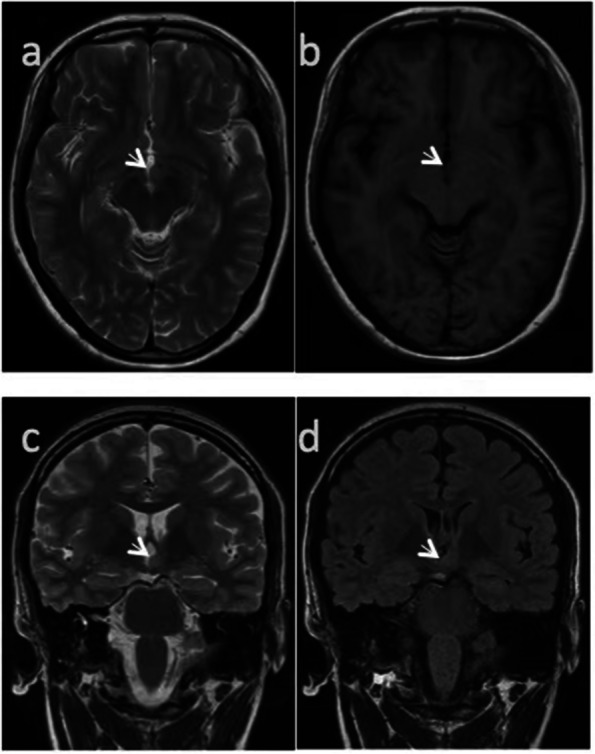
Cranial magnetic resonance imaging (MRI). Axial T2WI (a), T1WI (b), coronal T2WI (c), and T2 FLAIR (d) all showed presence of HH in the left side, with equal signal shadows. The HH had a size of 9 mm * 5 mm, and protruded into the third ventricle
